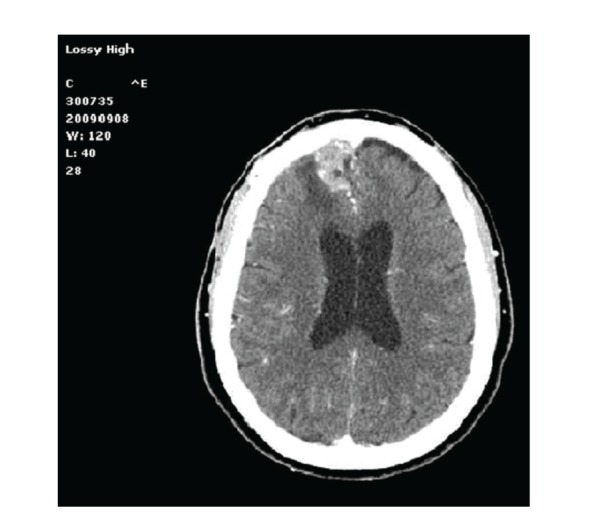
Contrast-enhanced axial CT scan of the postoperative intracranial granuloma.
